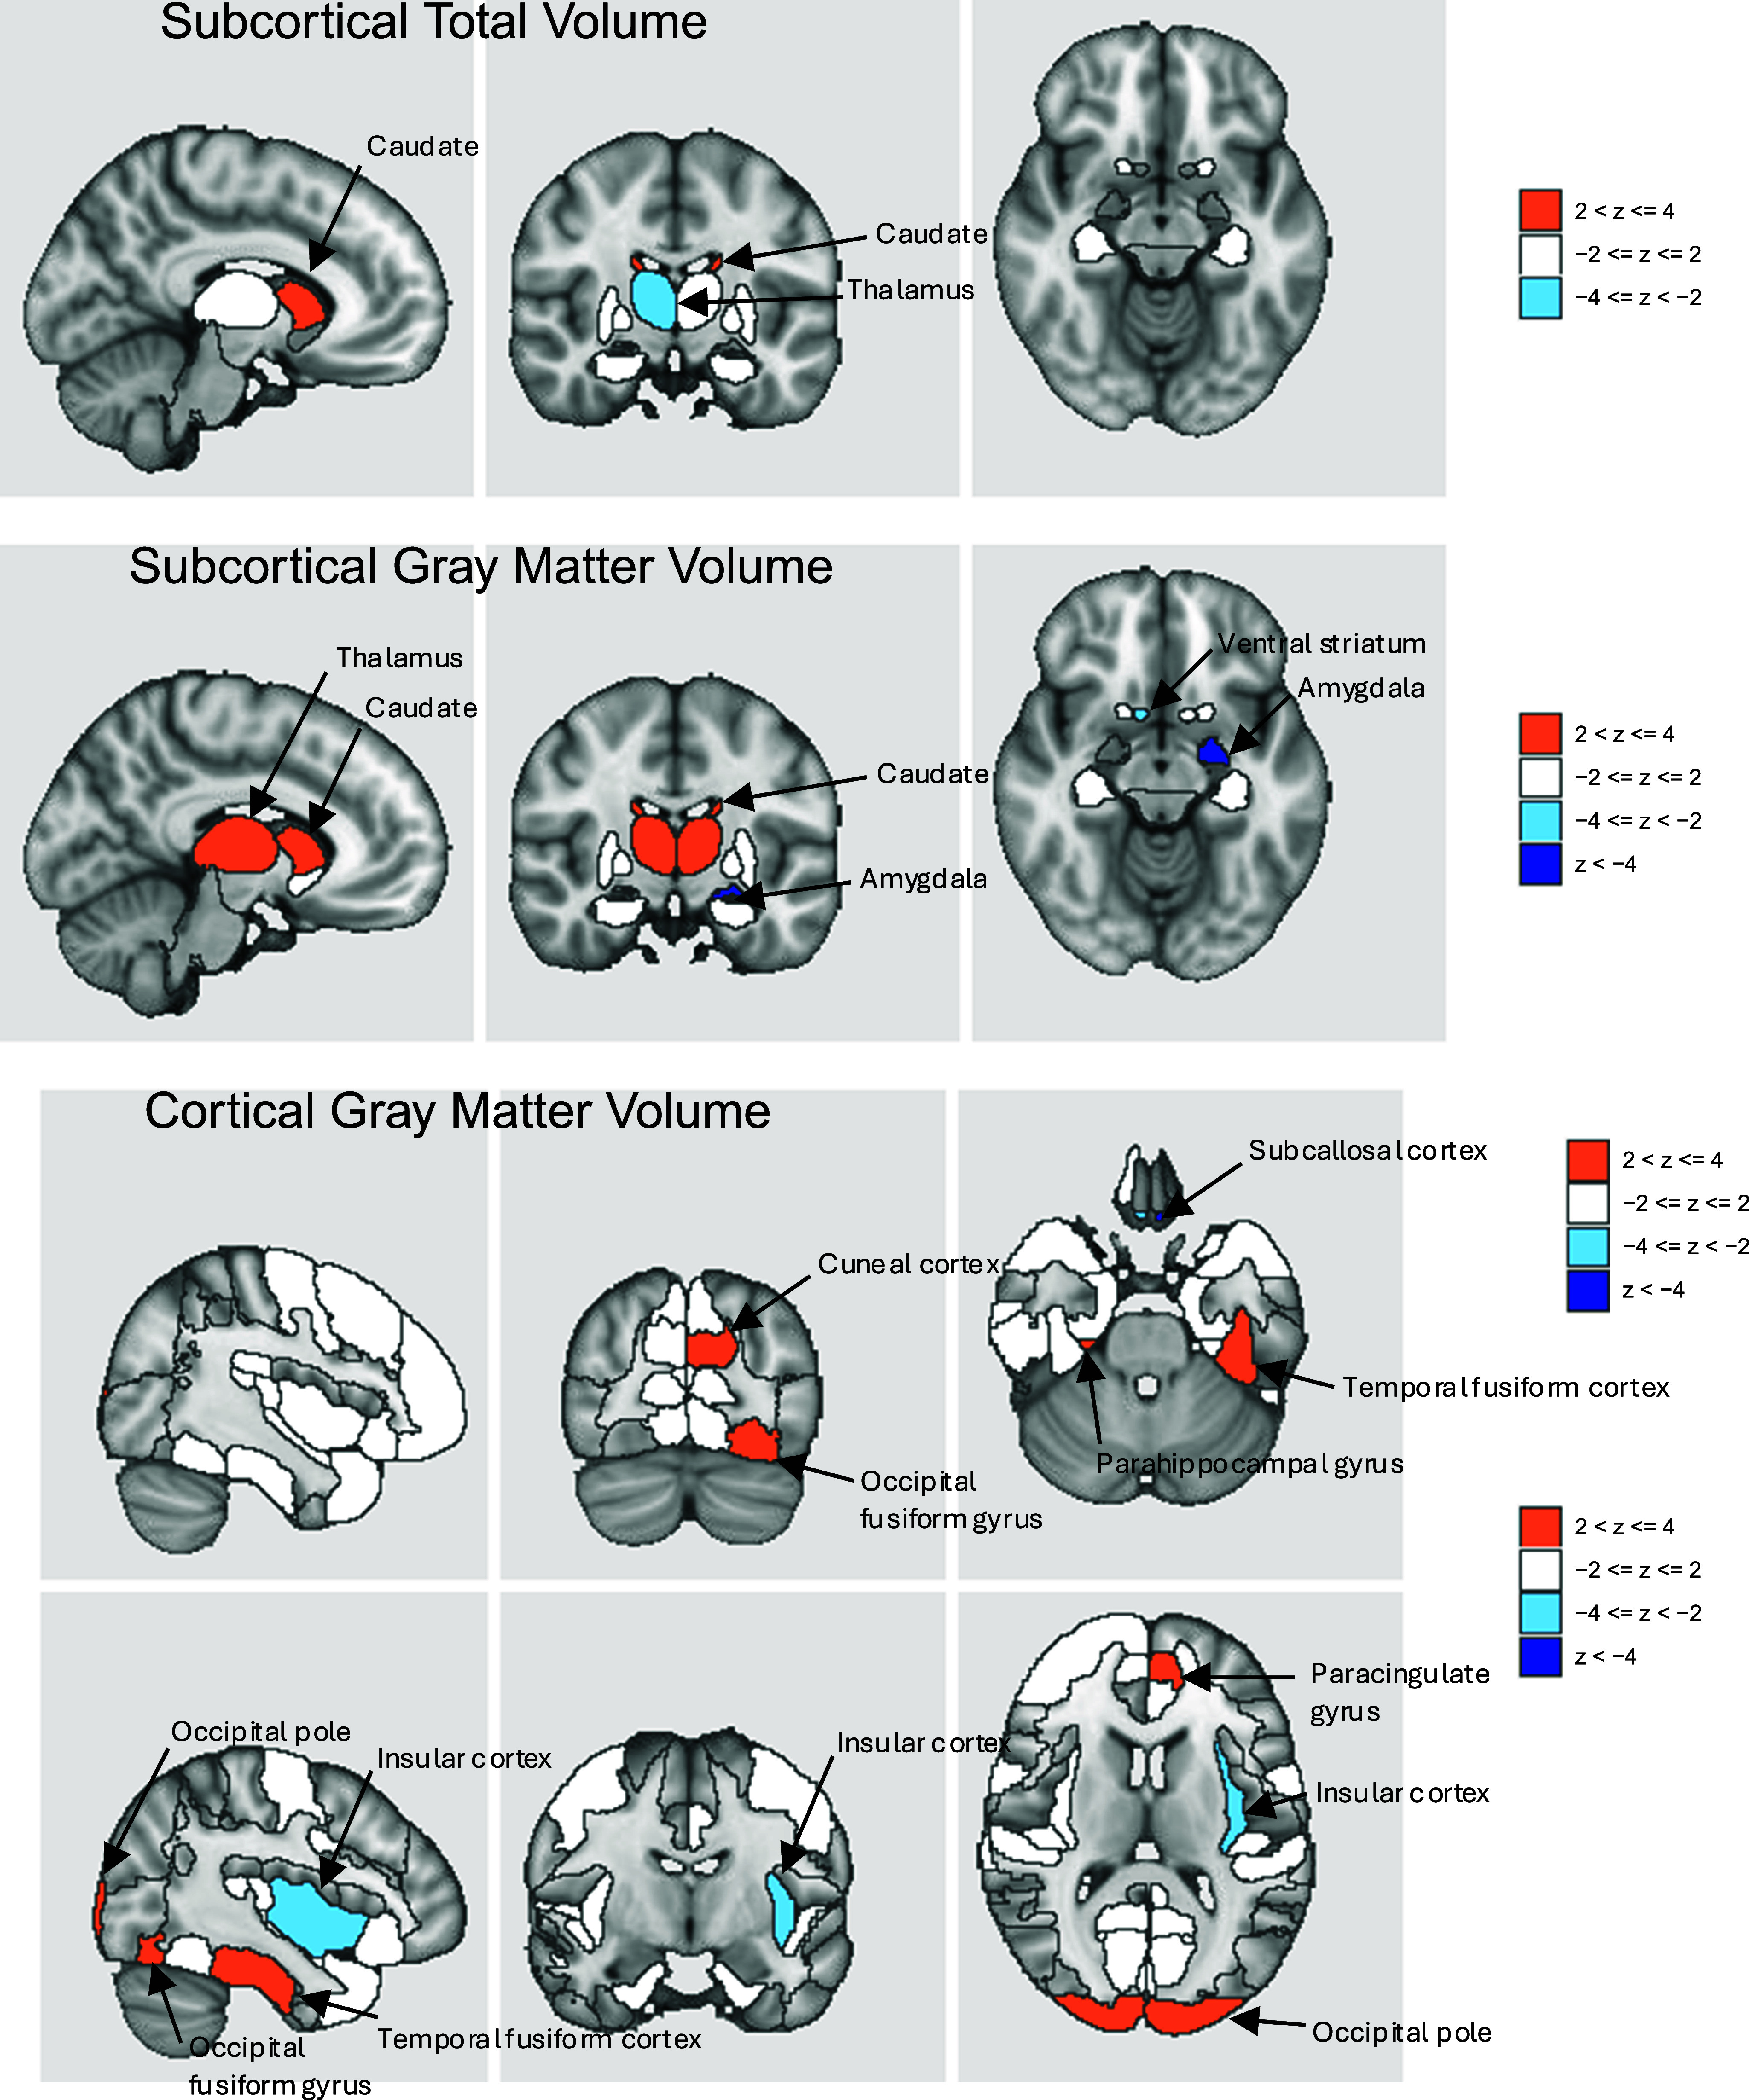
Brain region associations for the externalizing and internalizing (EXT + INT) factor. Associations shown are for image-derived phenotypes from structural magnetic resonance imaging. Full results are in Supplementary Figures 10–15 and Supplementary Tables 14–16. Blue colors represent reduced volume, and orange represents increased volume.
